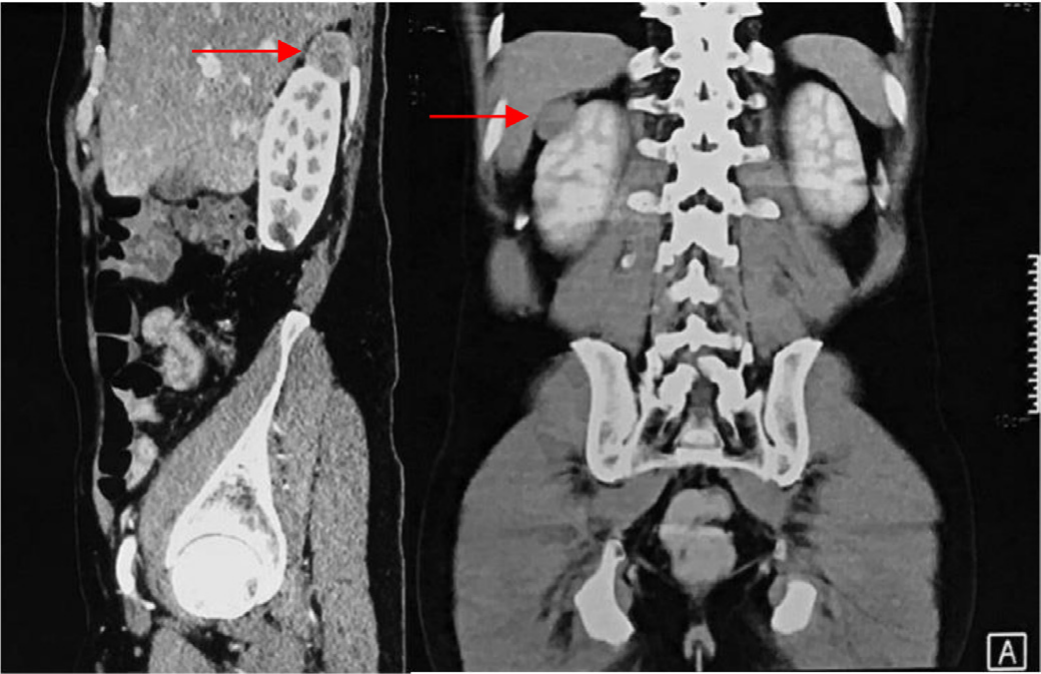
Display of the results of the CT abdomen examination.
